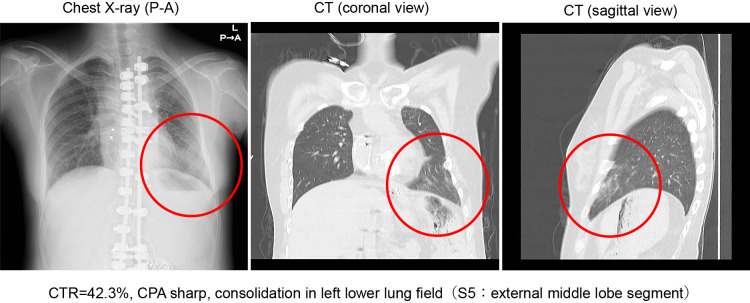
Chest X-ray and chest computed tomography imaging on admission Chest X-ray (posterior–anterior view) and chest computed tomography (coronal and sagittal views) reveal a consolidation in the left lower lung field (S5, external middle lobe segment). The cardiothoracic ratio (CTR) is 42.3%. CPA, costophrenic angle
